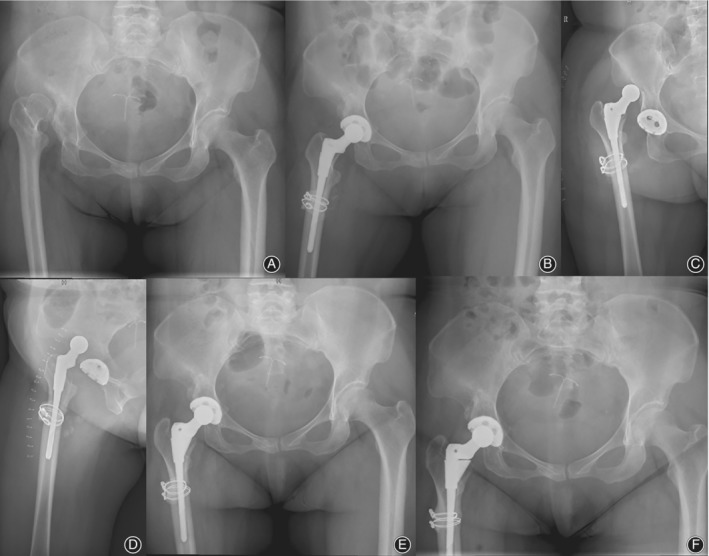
Radiographs of a 59‐year‐old woman with unilateral Crowe type IV DDH. (A) preoperative radiographic image showing high dislocation of the right hip. (B) Radiographic image at post‐operative day 1 showing that THA with a 22‐mm metal femoral head was performed in the right hip. (C, D) Dislocation occurred at post‐operative day 2. (E) Closed reduction was performed. (F) At the 5‐year follow‐up, no further dislocation or loosening of the component was identified.
